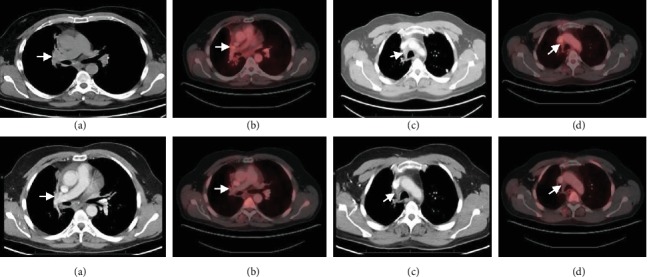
Top—CT and PET-CT images April 2012 before alisertib showing 2.1 cm R hilar mass (a) with SUV 4.8 (b) and R para-tracheal soft tissue prominence with fibrotic changes in previously irradiated field (c) with SUV 5.2 (d). Bottom—CT and PET-CT images December 2012 after 10 cycles of alisertib showing resolution of R hilar mass (a, b) and unchanged R para-tracheal cicatricial changes (c) with maximum SUV of 2.3 (d).
